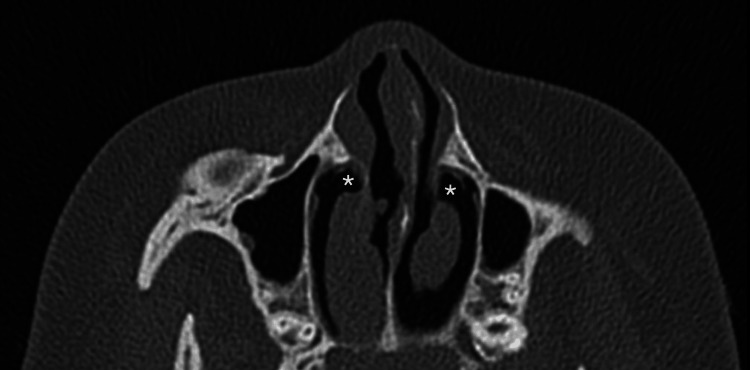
Axial view of the CT scan of the sinus showing the bilateral agenesis of the head of the inferior turbinates The asterisk represents the agenesis of the head inferior turbinate. CT: computed tompgraphy
